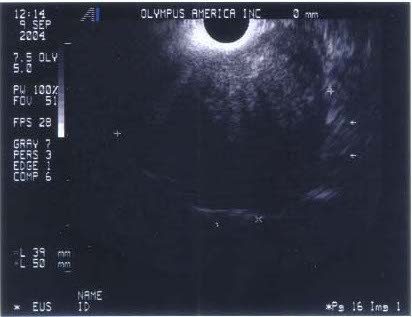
Endoscopic ultrasound image of a 52 x 39 mm hypoechoic mass in the upper mediastinum (Olympus UC-30P).
